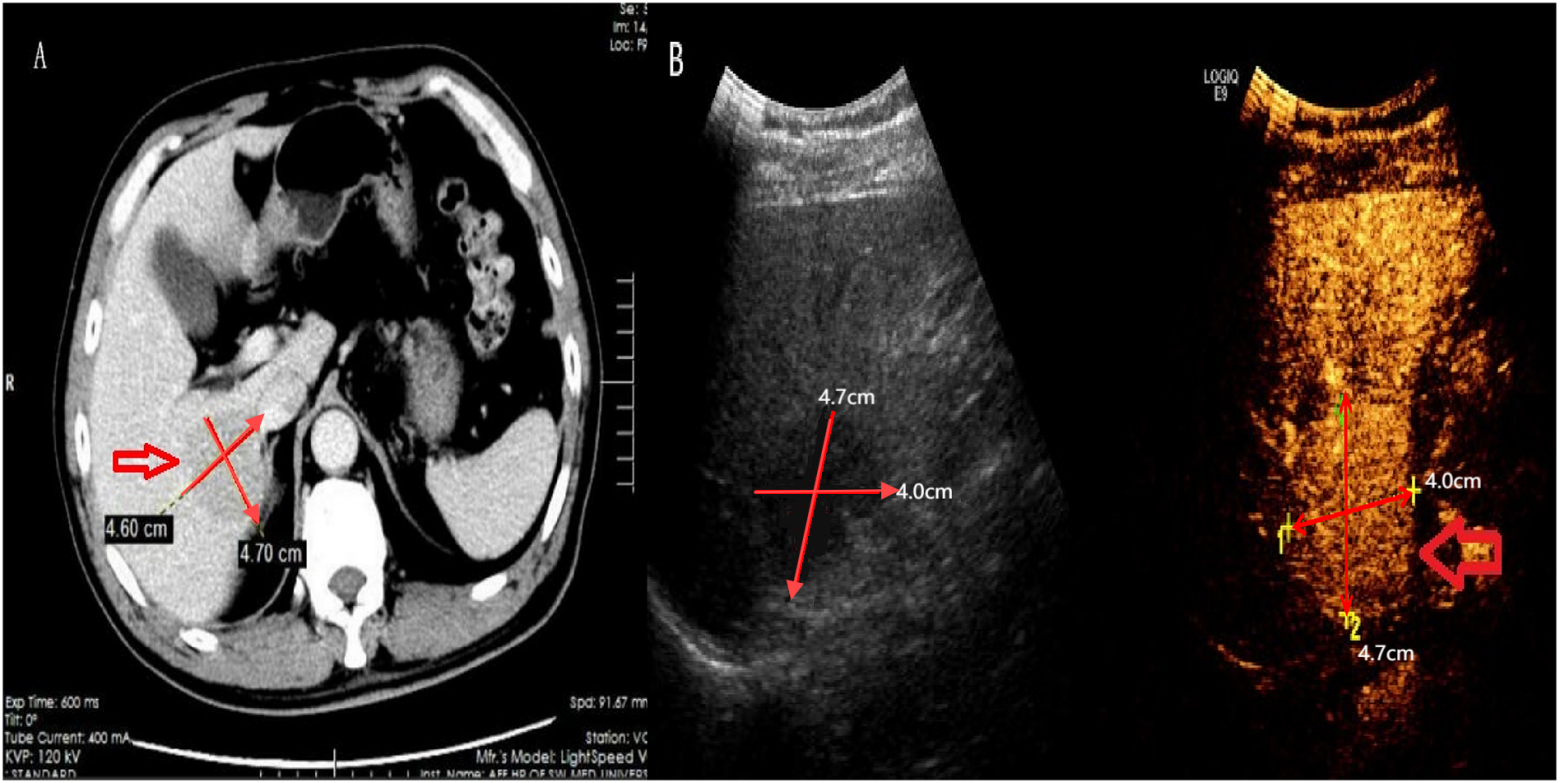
Computed tomography and contrast ultrasound results. Shown here the lump with hypodense lesion on CT (A) and low echo on contrast ultrasound and contrast-enhanced ultrasonography (B).
